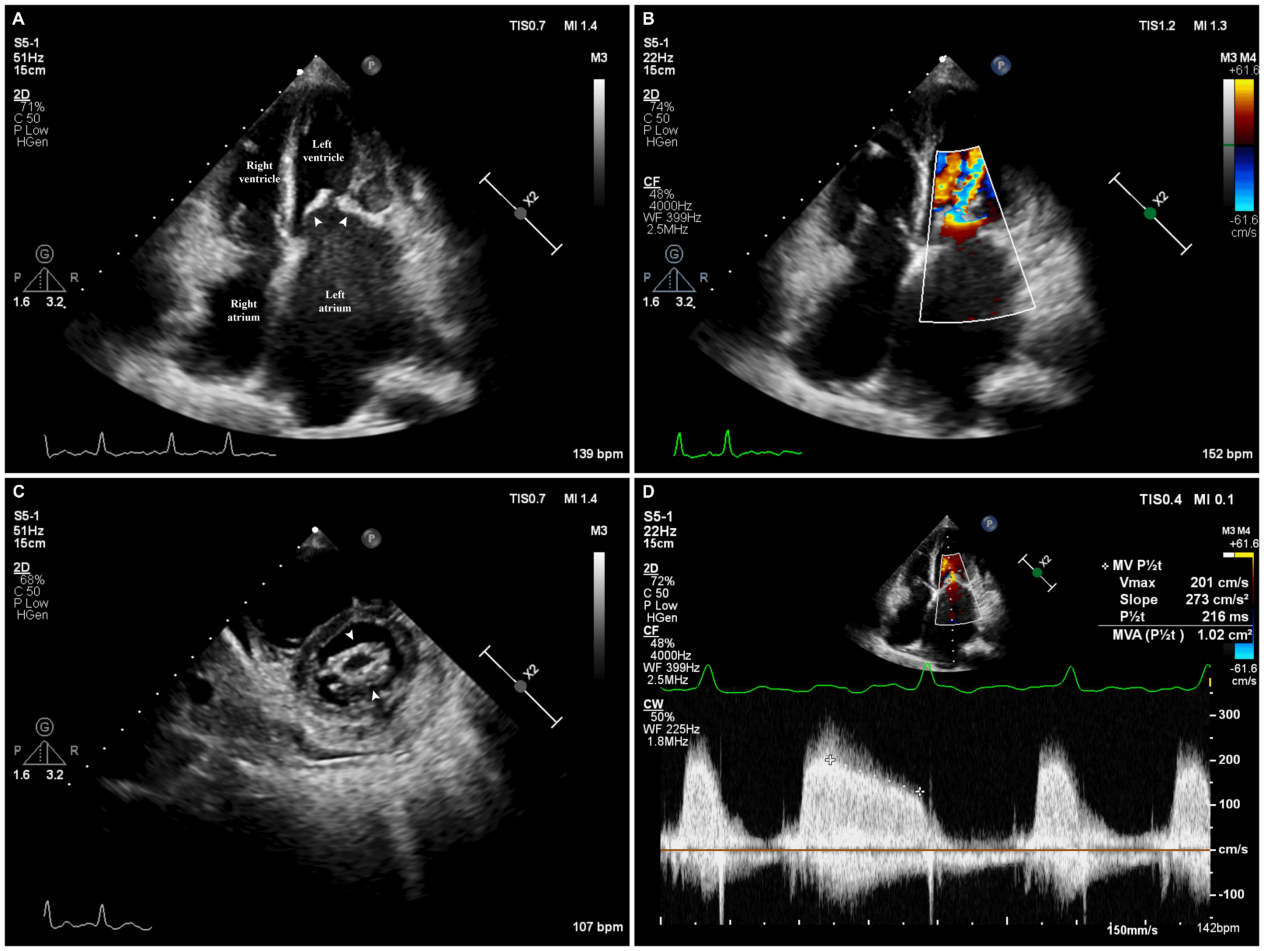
Severe mitral stenosis in a patient with shock (A) Apical four-chamber view demonstrates thickened mitral valve leaflets and a dilated left atrium (arrowheads). (B) Color Doppler imaging across the mitral valve shows turbulent flow across the mitral valve in diastole. (C) Parasternal short-axis view demonstrating thickened mitral valve leaflets (arrowheads) with commissural fusion giving a fish-mouth appearance. Mild pericardial effusion can also be appreciated. (D) Continuous-wave Doppler across the mitral valve demonstrates an elevated gradient. The valve area, as measured by pressure half-time, is 1 cm^2^. Image credit: Dinkar Bhasin
